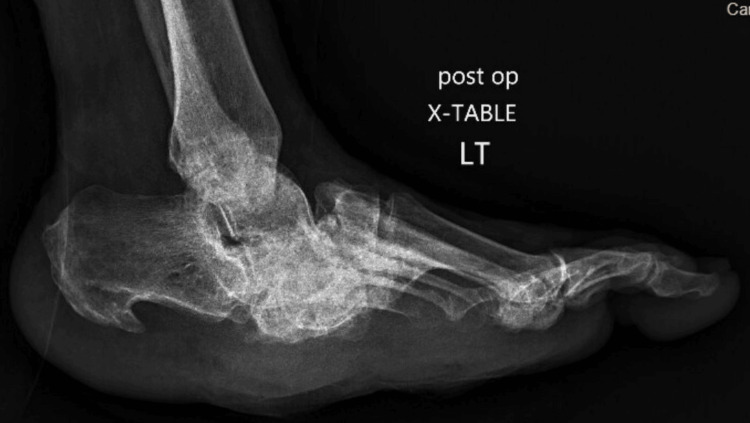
Postoperative lateral radiograph. Immediate postoperative non-weight-bearing lateral radiograph demonstrating resolution of the plantar exostosis after exostectomy, with realignment of the midfoot contour and offloading of the ulcer site.
